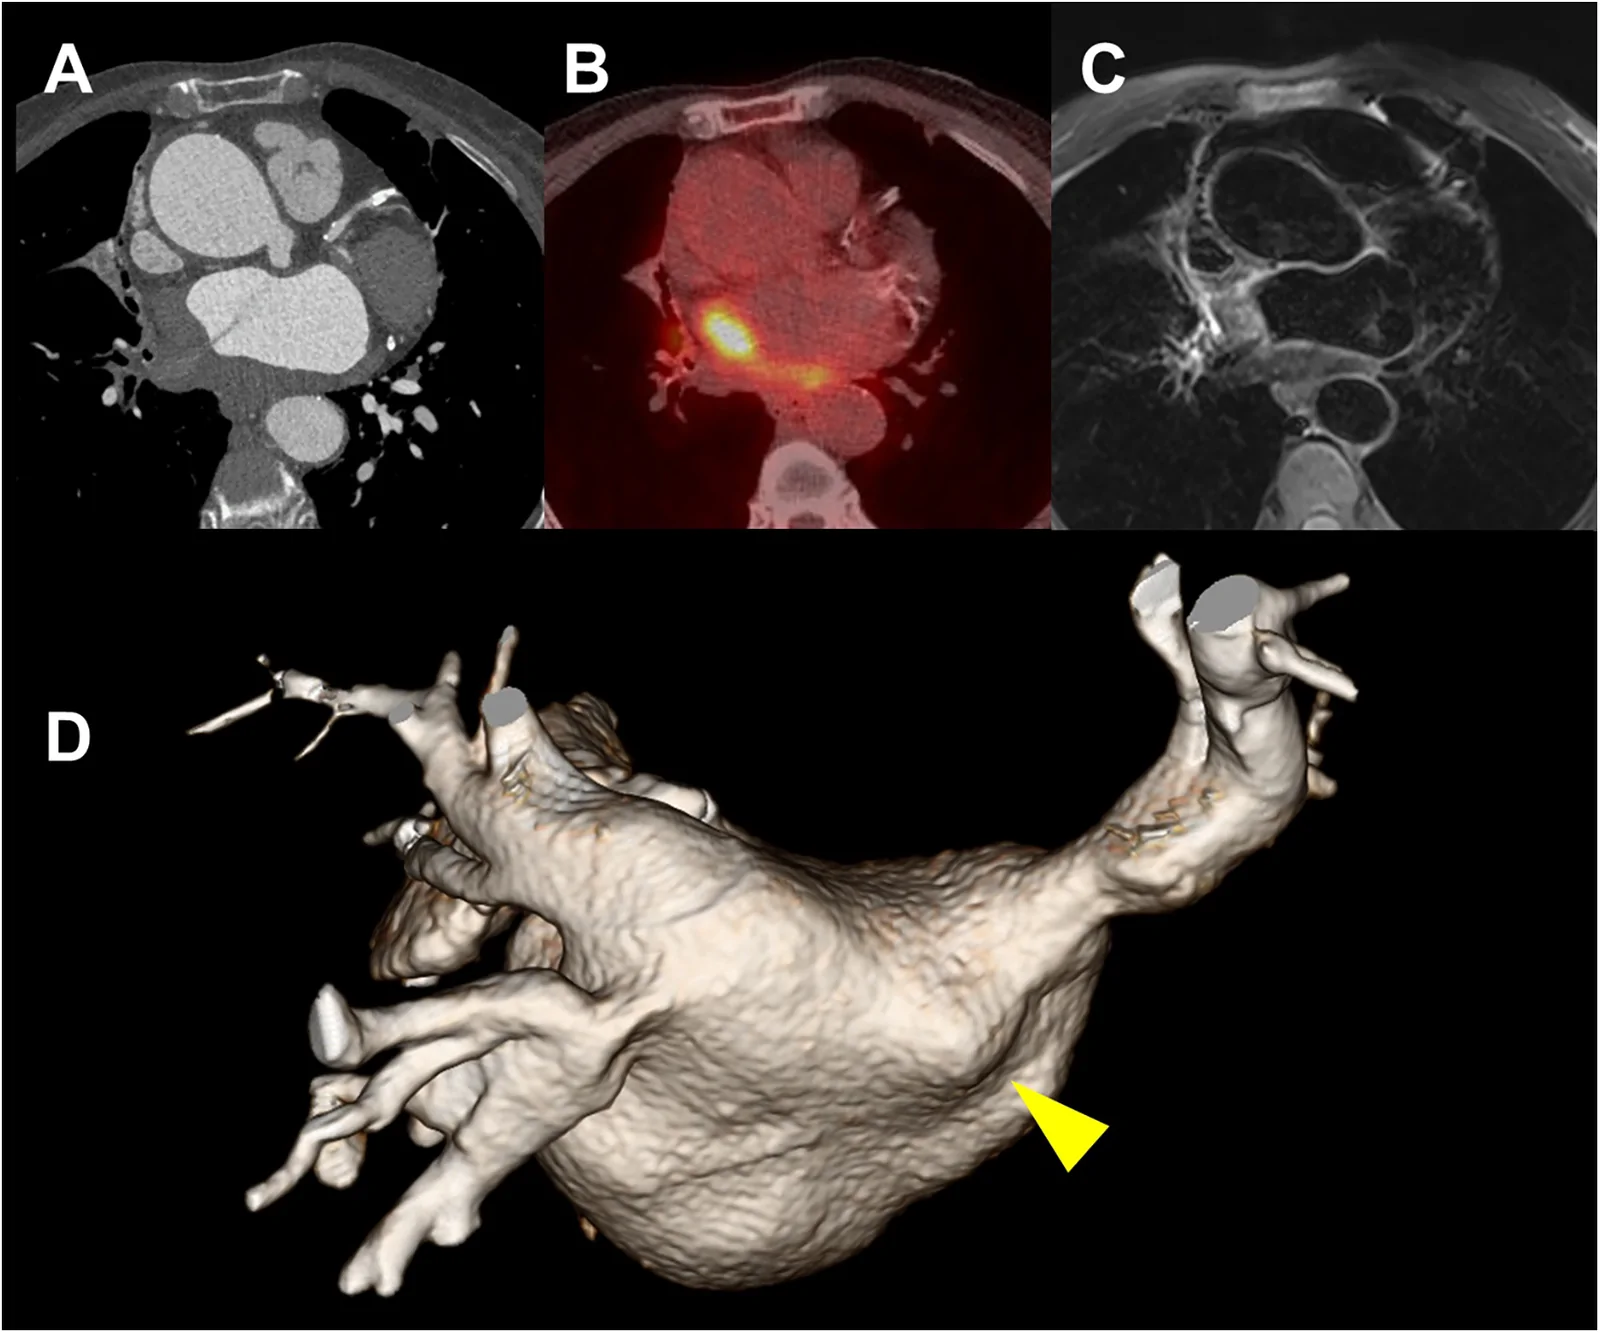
(A) and (D) cardiac computed tomography demonstrated the left atrial wall mass and obliteration (yellow arrow head) of right inferior pulmonary vein. (B) Torso PET-CT(F-18 FDG) showed heterogeneous hypermetabolic lesion in the left atrium posterior wall. (C) Axial black-blood T2-weighted magnetic resonance image showing a heterogeneous hyperintense left atrial mass, suggestive of a malignant cardiac tumor.
